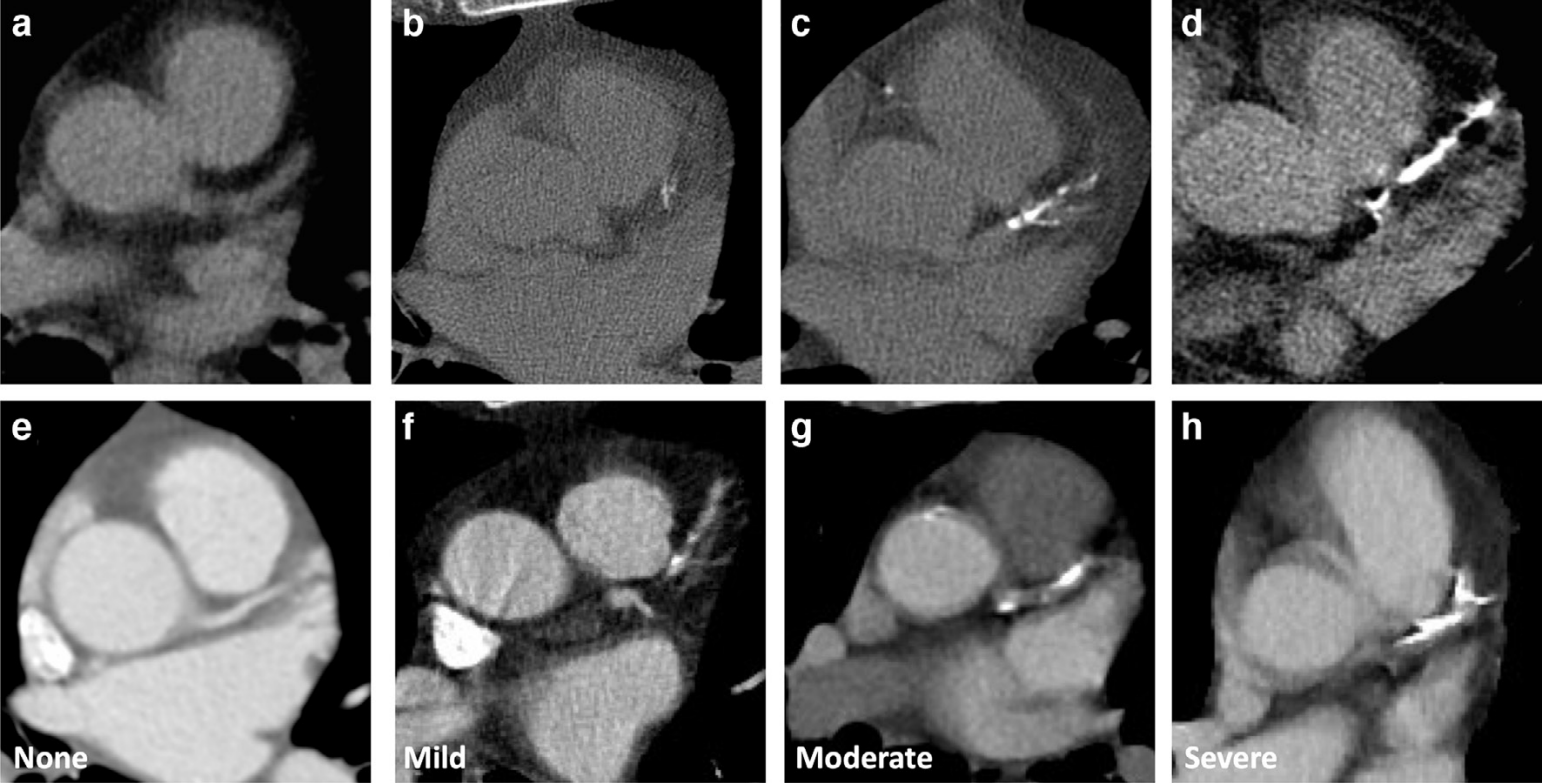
Coronary artery calcification severity. Images show different severity of coronary calcification from different patients on non-contrast (A–D) and contrast enhanced CT (E–H). Images show no coronary artery calcification (A, E) and mild (B, F), moderate (C, G) and severe (D, H) coronary artery calcification.
